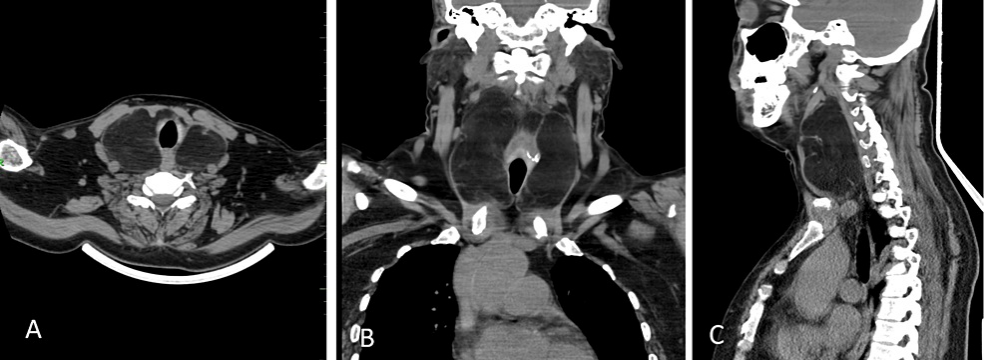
MRI findings A, B, and C: MRI of the neck showing a homogenously low-density mass of the neck with retrosternal and hypopharyngeal extension and bilateral displacement of the carotid sheath.
